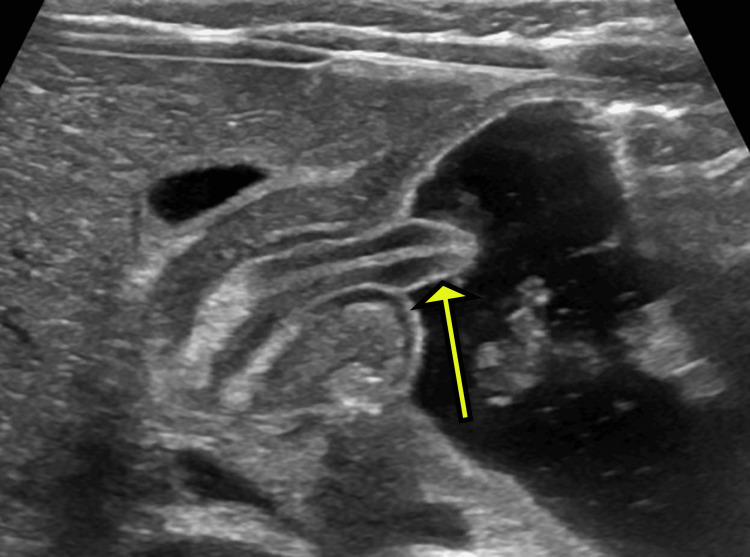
Longitudinal ultrasonography of the antropyloric region shows that the antrum is distended by fluid and the mucosa from the pylorus is squeezed out into the antrum, giving the "nipple" appearance (yellow arrow).
